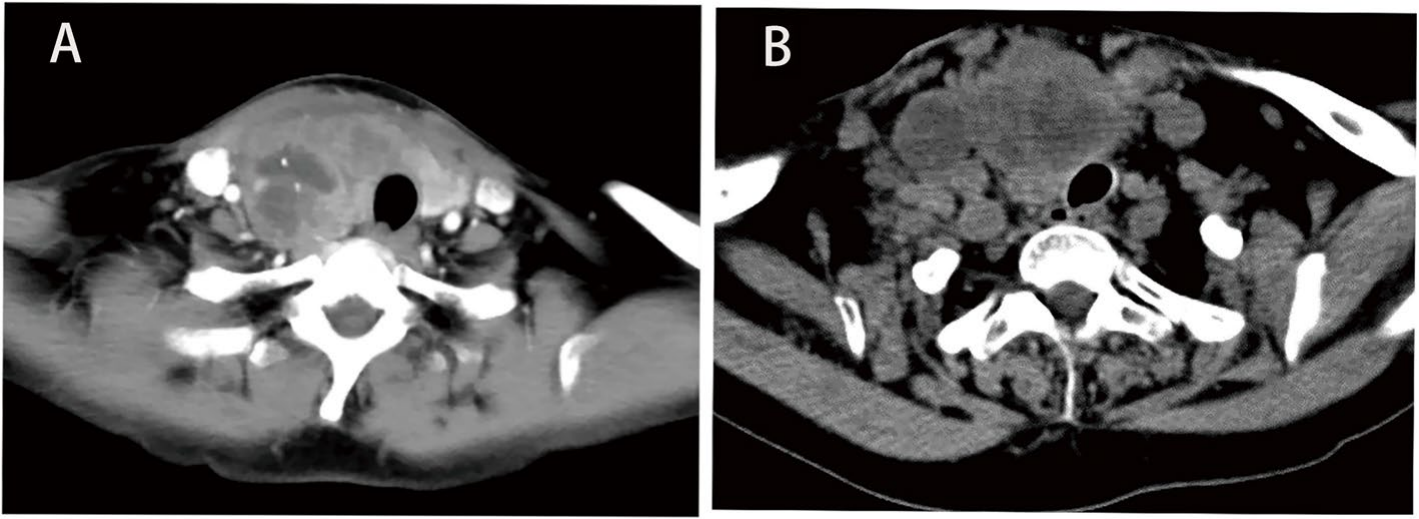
Axial CT image of the neck shows a large right thyroid mass with trachea compression in (A) and (B), respectively
